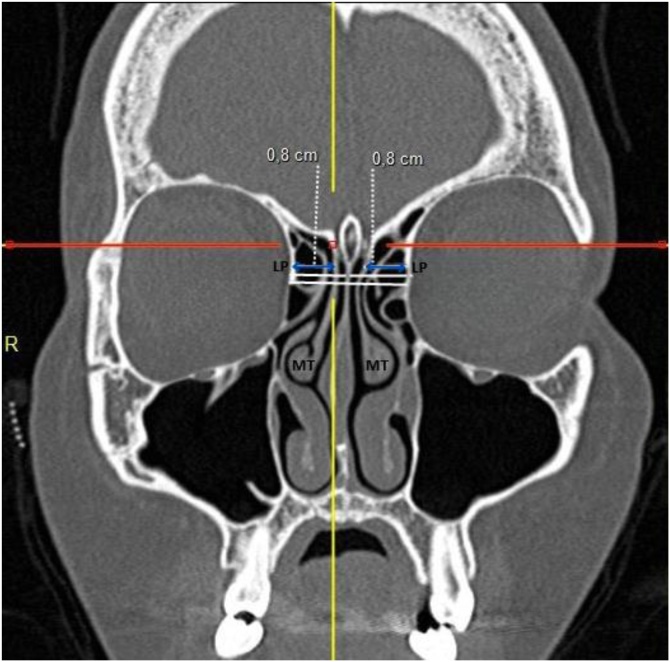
Coronal CT view showing the distance between the Lamina Papyracea (LP) and the vertical portion of the Middle Turbinate (MT), representing the lateral boundary of the frontal recess for Draf type IIA procedures.
